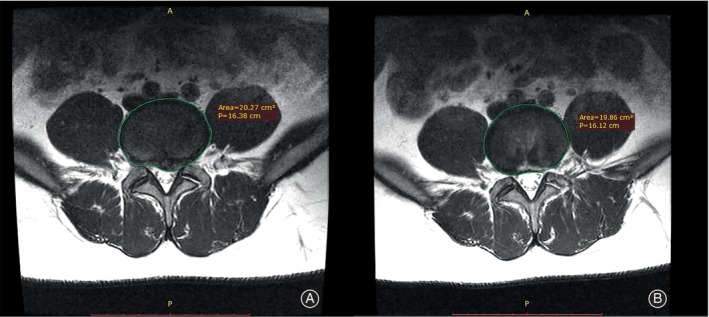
A 29‐year‐old patient is shown preoperatively and postoperatively at the same lumbar spine MRI cross‐section level. The disc area preoperatively was 20.27 cm^2^ and postoperatively was 19.86 cm^2^. The changes in VAS‐B at 3, 6, and 12 months postoperatively were 3, 3, and 3. Those in VAS‐L at 3, 6, and 12 months postoperatively were 8, 9, and 9.
